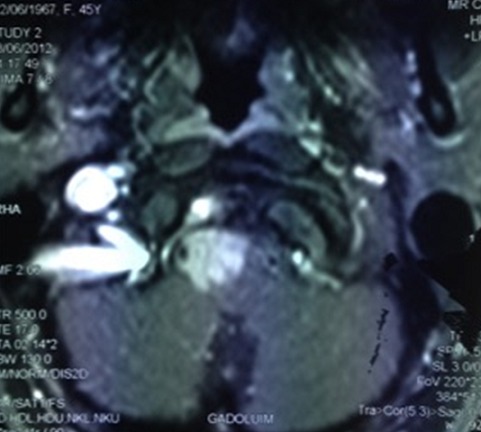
Axial T1 weighted MR image shows an anterolateral foramen magnum meningioma
